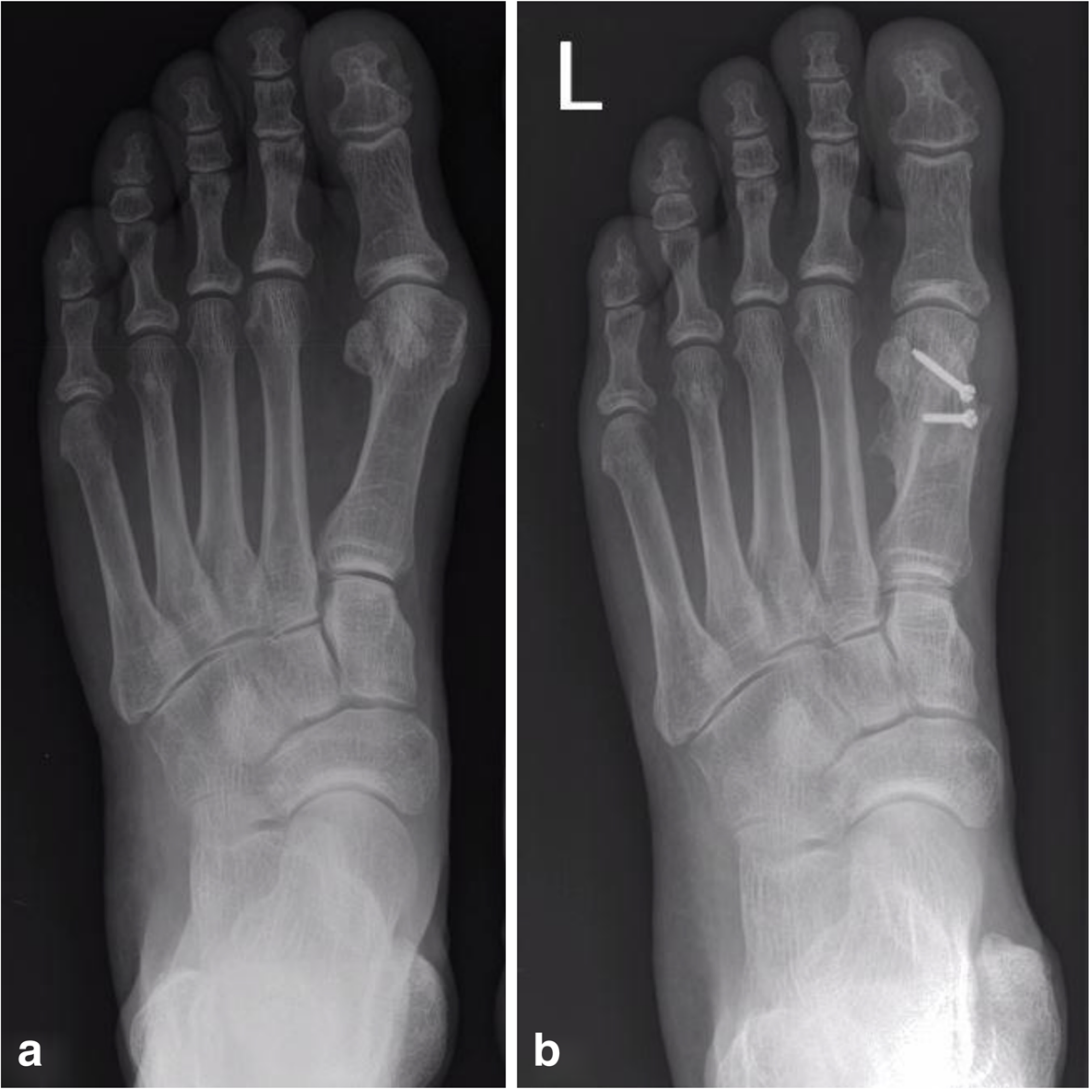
Conventional dorsoplantar radiographs of the left foot in a 60-year-old male. a Preoperative radiograph showing a hallux valgus deformity. b Postoperative radiograph showing correction of hallux valgus deformity 1 year after reversed L-shaped osteotomy (ReveL) using two screws
